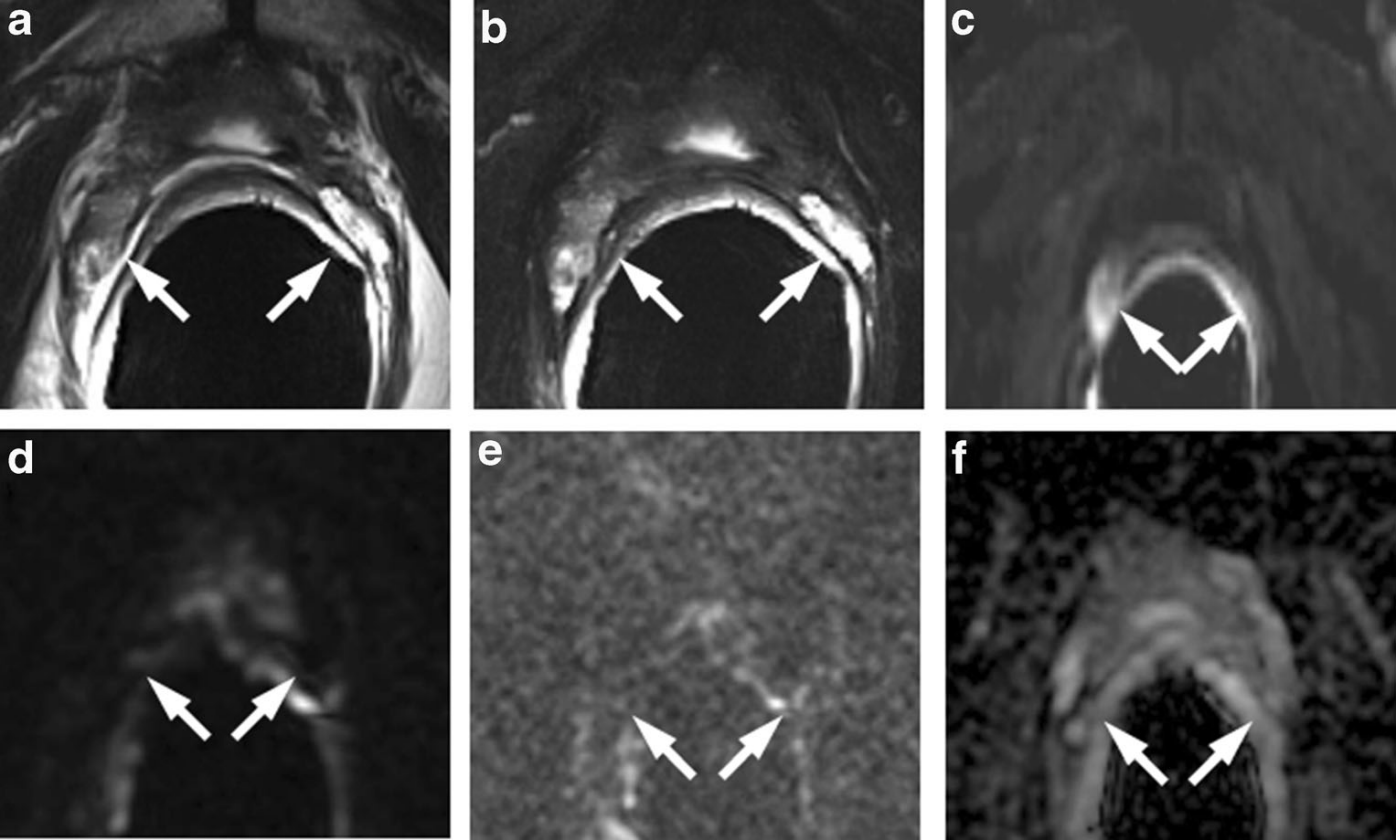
a Axial T2-weighted fast spin-echo (6330/115) and b axial T2-weighted fat saturated fast spin-echo image (8450/115) show, on the zone previously occupied by the right seminal vesicle, a slightly hyperintense lobulated tissue. c Gradient-echo T1-weighted subtracted image shows a well-defined area of marked enhancement (arrows) on the same location as the lobulated tissue seen on T2-weighted images. d Diffusion weighted image with a b value of 1.000 and *e* 3.000 s/mm^2^ and f the ADC map show no restricted diffusion signs. These findings are consistent with prominent periprostatic venous plexus (*white arrows*)
